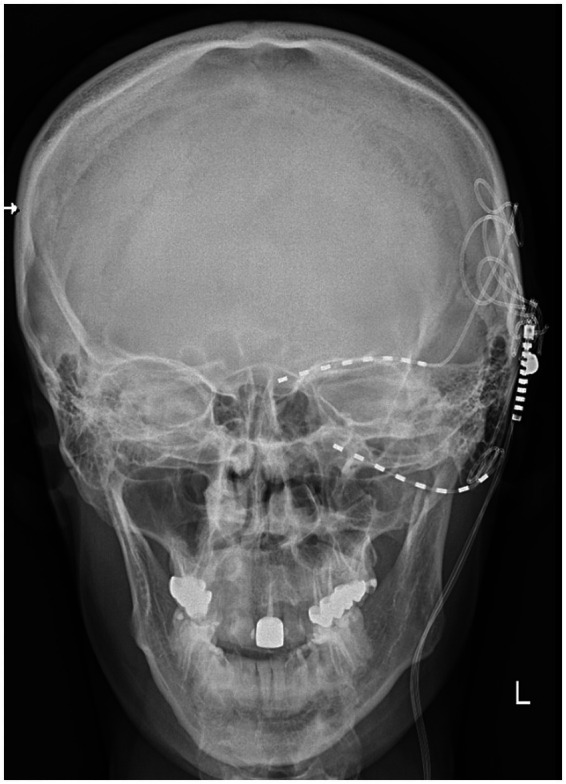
X-ray image showing the additional electrode lead positioned above the eyebrow for supraorbital nerve stimulation and the electrode lead in the pterygopalatine fossa in the patient (MM).
